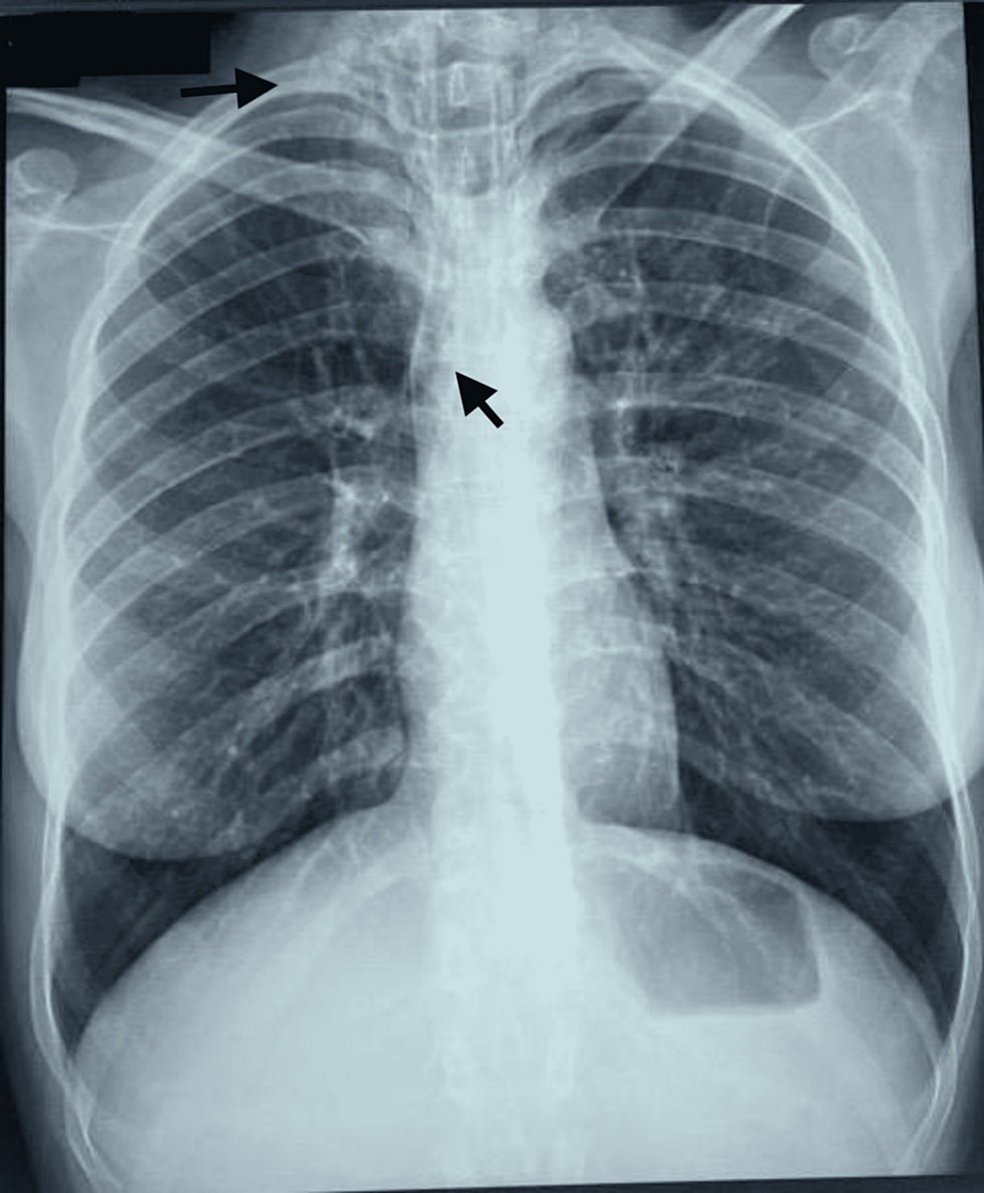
Posteroanterior chest radiograph demonstrates typical features of pneumomediastinum. The image shows aerial border along the edge of the cardiac silhouette associated with subcutaneous hyperclarities of the cervical region, drop heart appearance, and thoracic distension.
